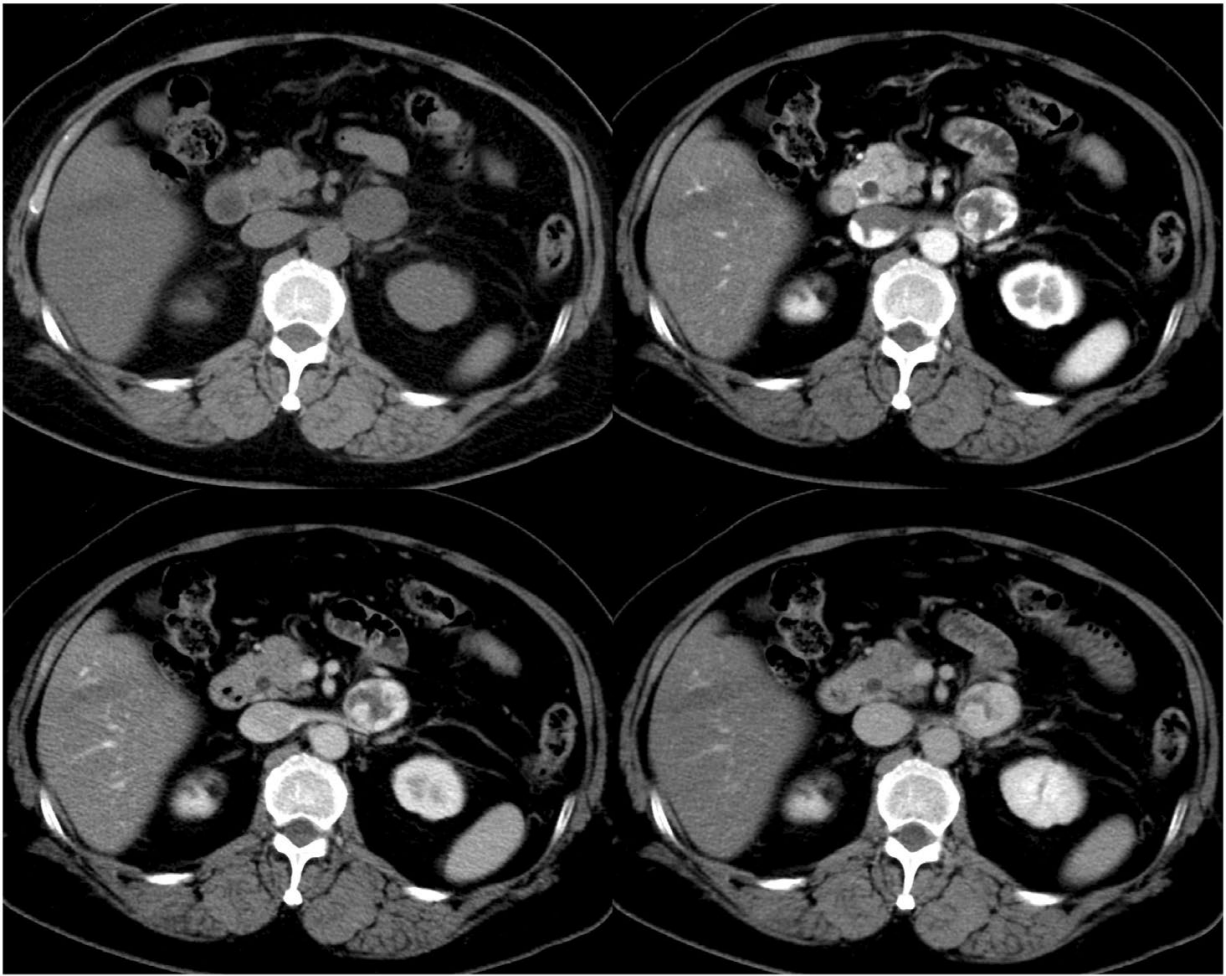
Abdominal CT imaging at the initial diagnosis. CT imaging reveals a 31 × 26 mm gradually heterogeneous enhanced tumor in the retroperitoneal area that does not invade the surrounding tissue.
